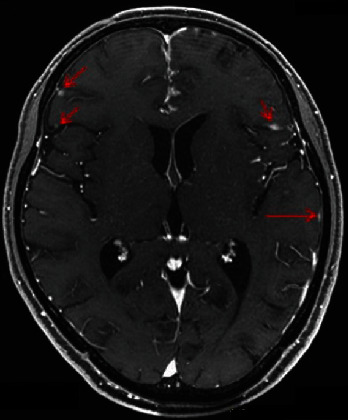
T2-weighted magnetic resonance imaging revealed multiple leptomeningeal metastases at bilateral trigeminal, facial, and abductor nerves and surrounding tissues of the brain stem and cervical spinal cord (arrows).
